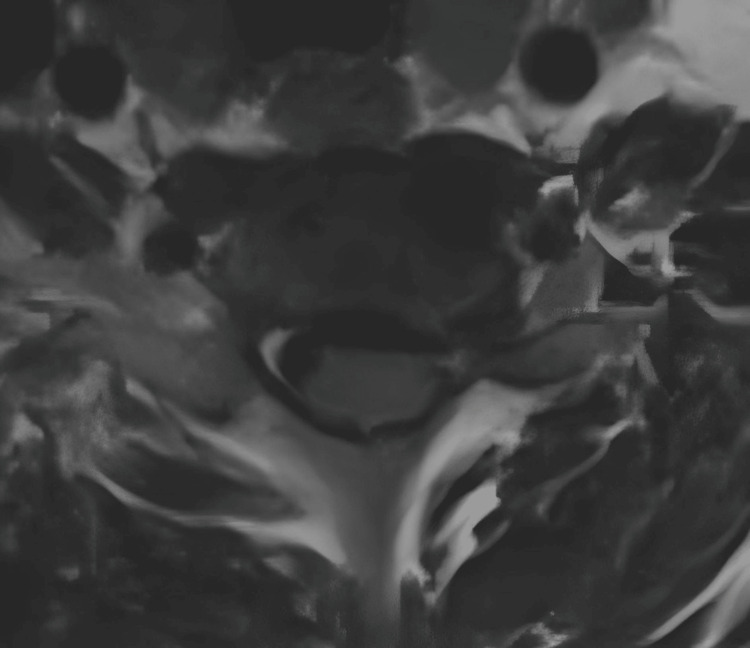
Patient's preoperative magnetic resonance imaging (MRI) The cross - section of the patient's cervical spine MRI shows that the intervertebral disc protrusion compresses the dura mater and nerve roots, especially in the left intervertebral foramen area, which is more obvious.
